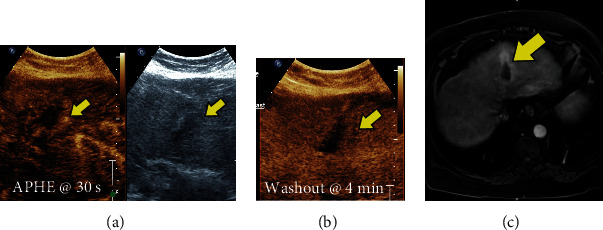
(a) Contrast-enhanced US image (left) and grayscale US image (right) confirming arterial enhancement (arrow) around ablation cavity questioned on (c, arrow) MRI. (b) Delayed washout is also visualized on CEUS at 4 min (arrow) consistent with residual HCC. (c) Axial T1 weighted postcontrast MRI image through the upper abdomen showing contrast enhancement around the ablation cavity (arrow).
